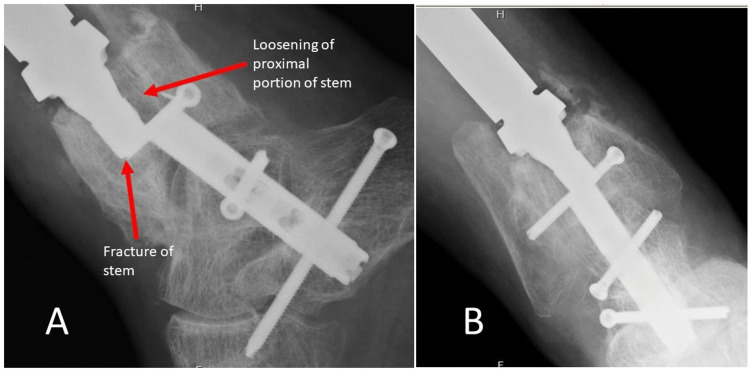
Radiographs of the failed stem (A, B).
